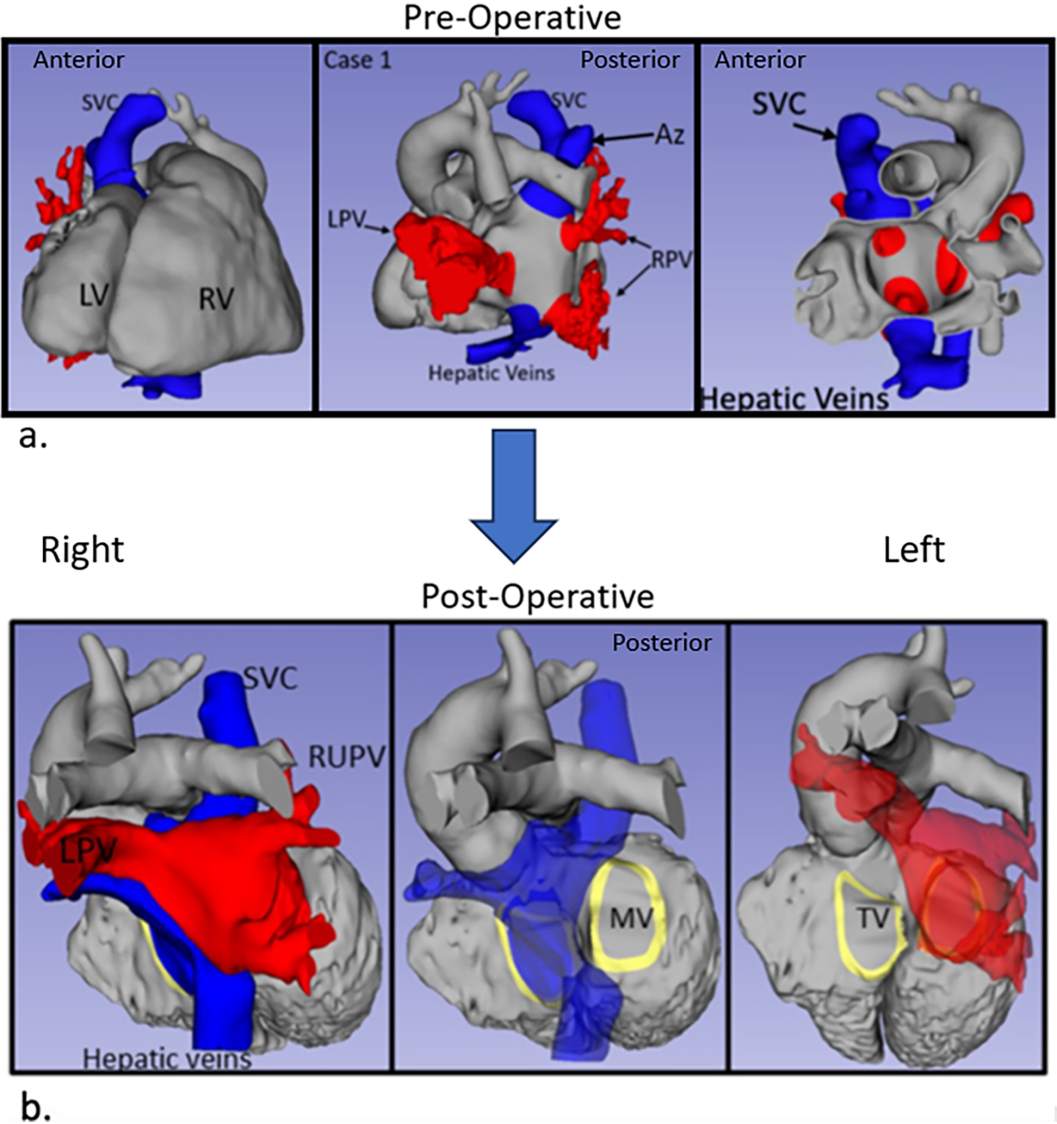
3D renderings derived from MRI in Case 1. (a) Pre-operative anatomy views shown from left to right: anterior, posterior, and anterior slice through the atria. (b) Post-operative anatomy. Systemic veins are colored blue and pulmonary veins are red. Az, azygous vein; LV, left ventricle; LPV, left pulmonary veins; RPV, right pulmonary veins; RV, right ventricle; SVC, superior vena cava.
